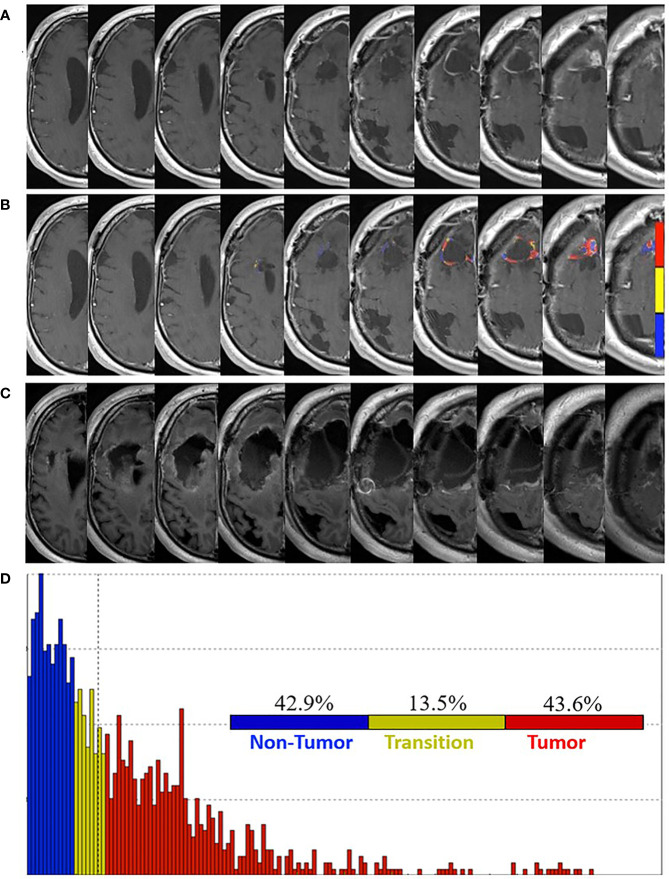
Pre- and post-surgery MRI. (A) Postcontrast T1-weighted MRI obtained 20 weeks post-pLDR and 10 weeks before redo-surgery. (B) Corresponding maps of FTB superimposed on the postcontrast T1-weighted MRI images. The FTB maps show areas of active tumor (red), treatment effect (blue), and transitional zone (yellow). (C) Immediate post-operative postcontrast T1-weighted MRI with FLAIR demonstrating extent of resection, which encompasses regions of tumor identified in the FTB maps. (D) Distribution of T1-weighted enhancement volume based on vascularization as measured by FTB maps.
